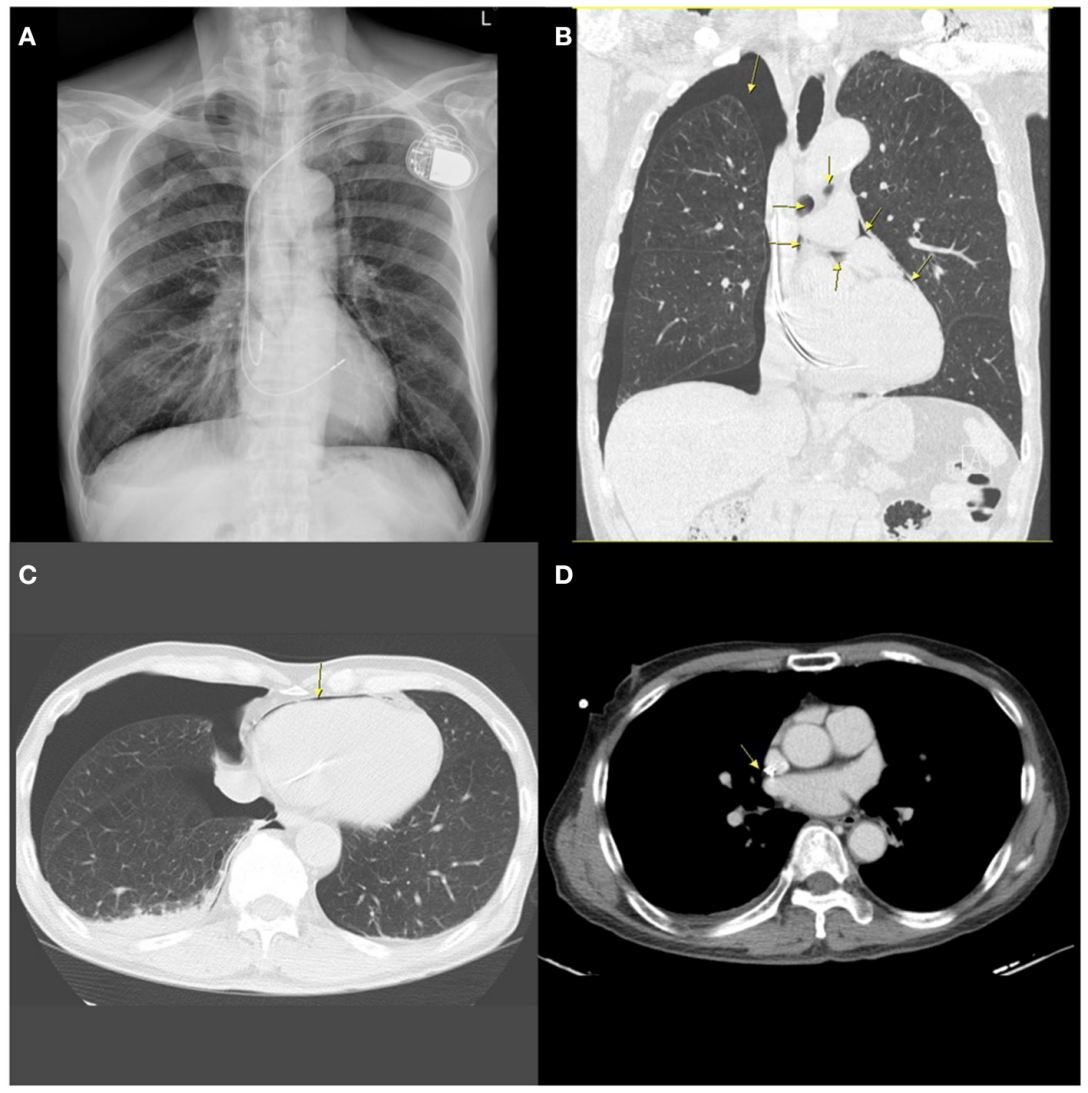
A chest radiograph performed 5 h after implantation showing contralateral pneumothorax, pneumopericardium, and pneumomediastinum. (A) Posterior-anterior (PA) chest X-ray demonstrating contralateral pneumothorax and mild pneumomediastinum. (B) Coronal chest non-contrast computed tomography (CT) scan image showing right-sided pneumothorax, pneumomediastinum, and pneumopericardium (arrows). (C) Horizontal CT image showing pneumopericardium (arrow) and pneumothorax. (D) Horizontal slices of CT scan suggesting possible extrusion of the atrial lead through the right atrium (arrow).
